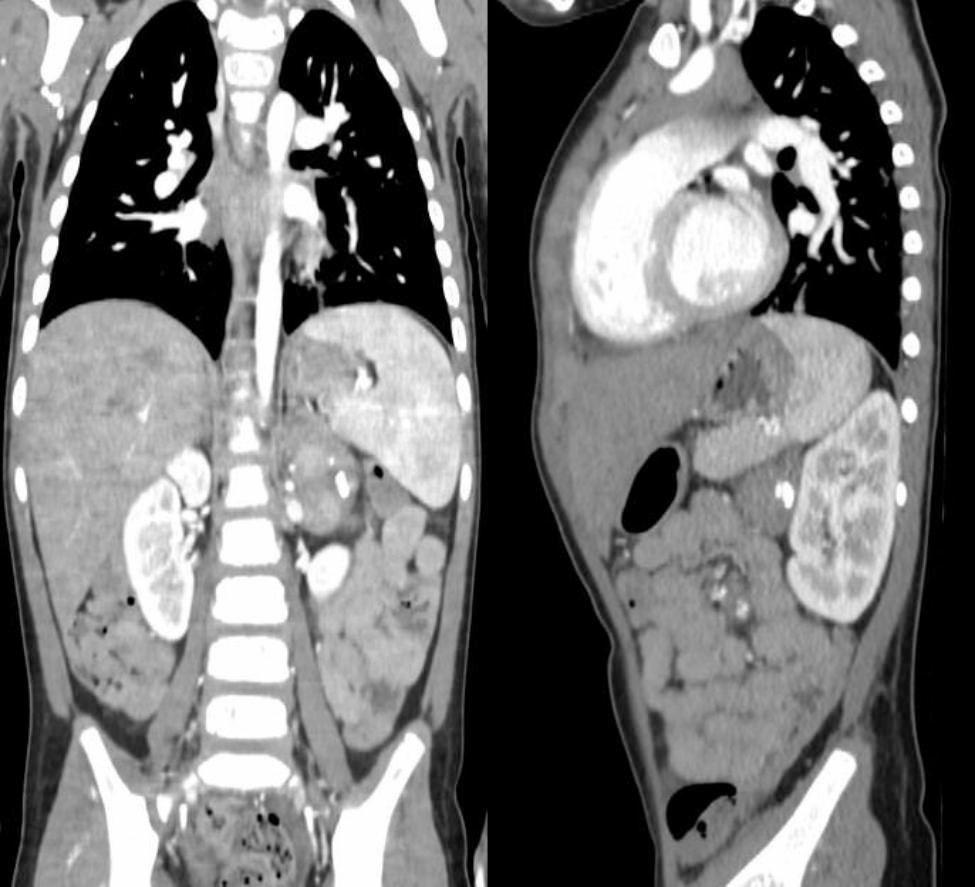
CT scan shows a solid, heterogeneous predominantly hypodense surrenal mass with eccentric calcification of dimensions of 2.5 cm x 2.7 cm x 4.5 cm
